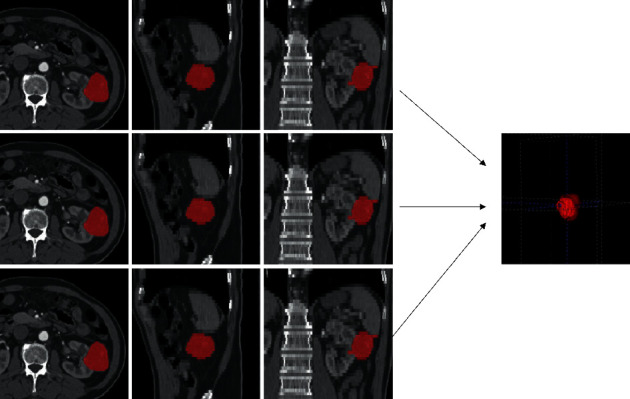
Computed tomography (CT) image segmentation in a corticomedullary phase enhanced CT scan.
